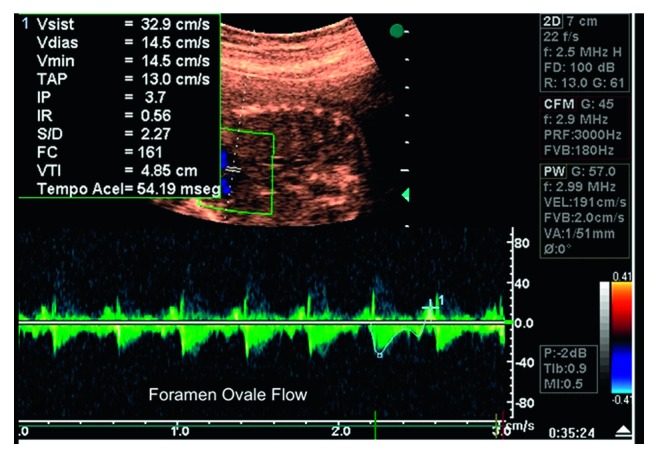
Foramen ovale flow and pulsatility index assessment: echocardiography of fetus at 33 weeks with intrauterine growth restriction. The foramen ovale pulsatility index is 3.70.
